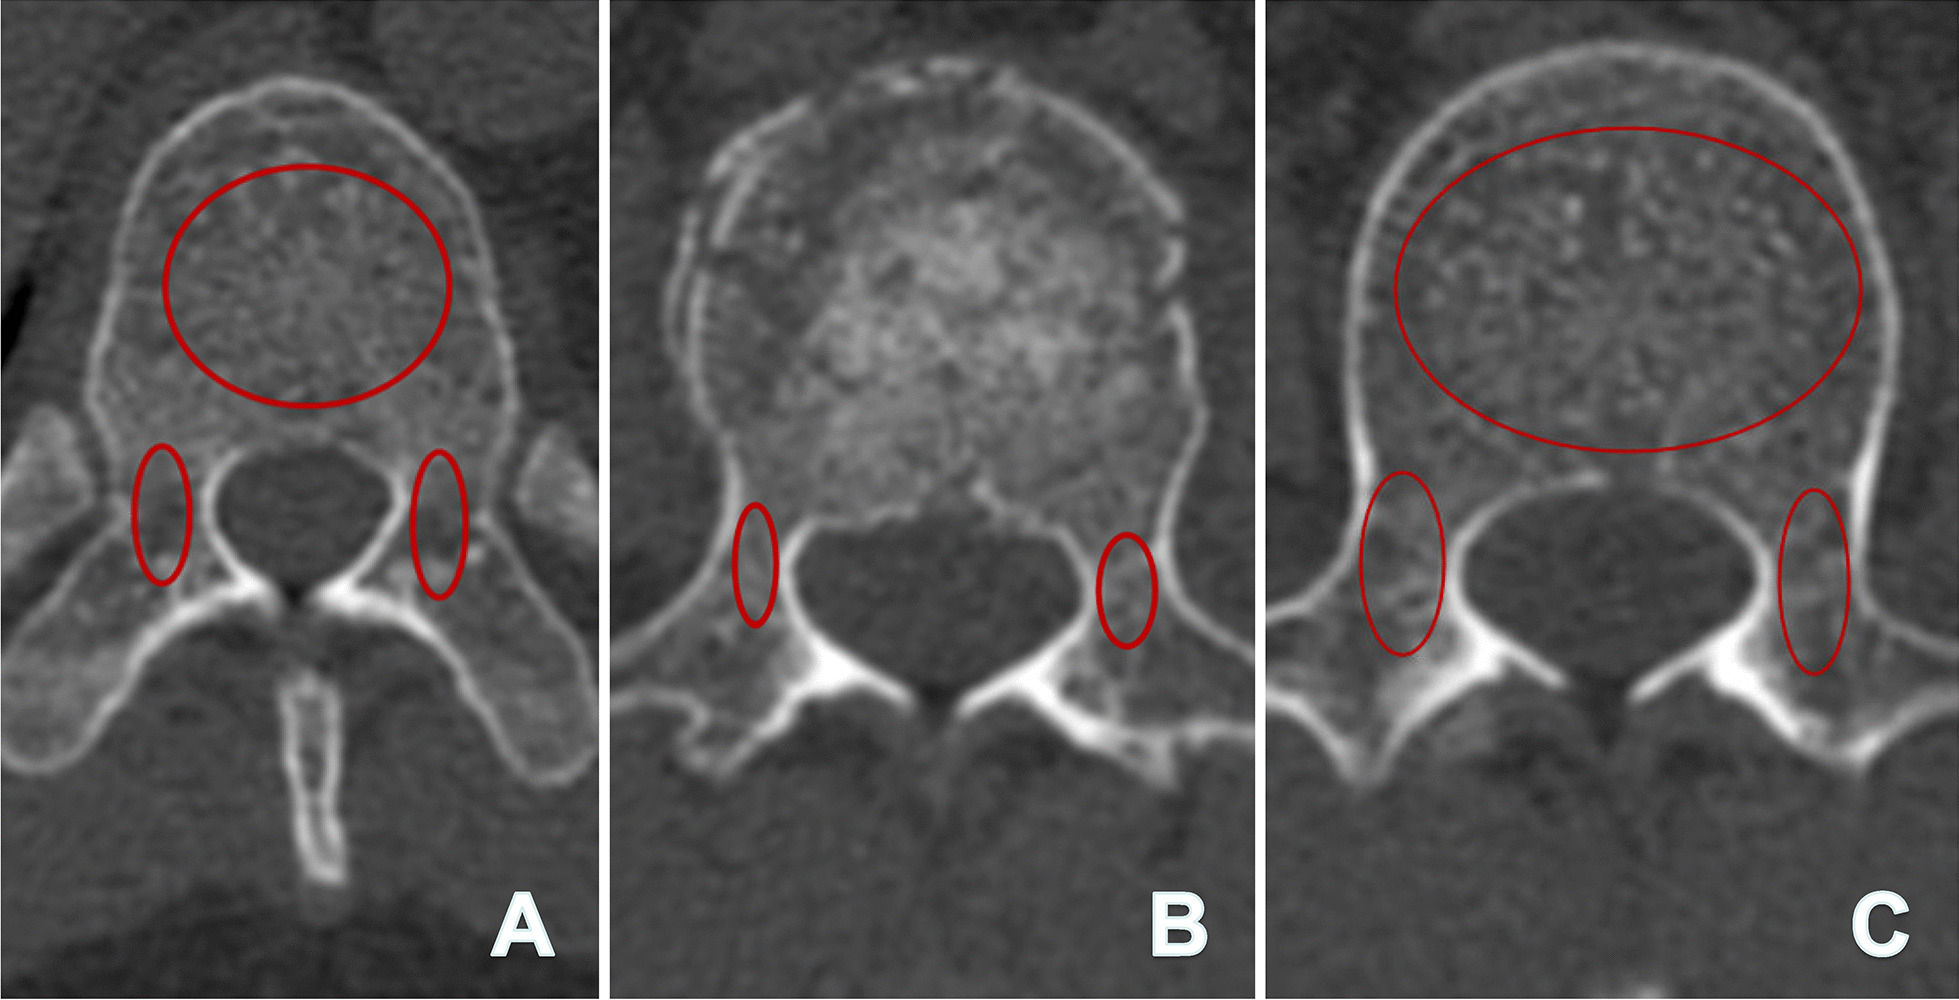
Measurement methods for mean CT Hounsfield Unit (HU) values: a 41-year-old male patient was diagnosed with a vertebral fracture in L1. A The mean CT Hounsfield Unit (HU) values of the T12 vertebral body (VB) were 176, and the right and left pedicles (excluding the cortical bone) were 133 and 136 respectively; B the mean HU values of the right and left pedicles of the L1 (fractured vertebra) were 157 and 144 respectively; C the mean HU values of the L2 VB was 164, and the right and left pedicle were 160 and 147 respectively. The mean HU values of the patient was 152.1 = [(176 + 133 + 136 + 157 + 144 + 164 + 160 + 147)/8]
